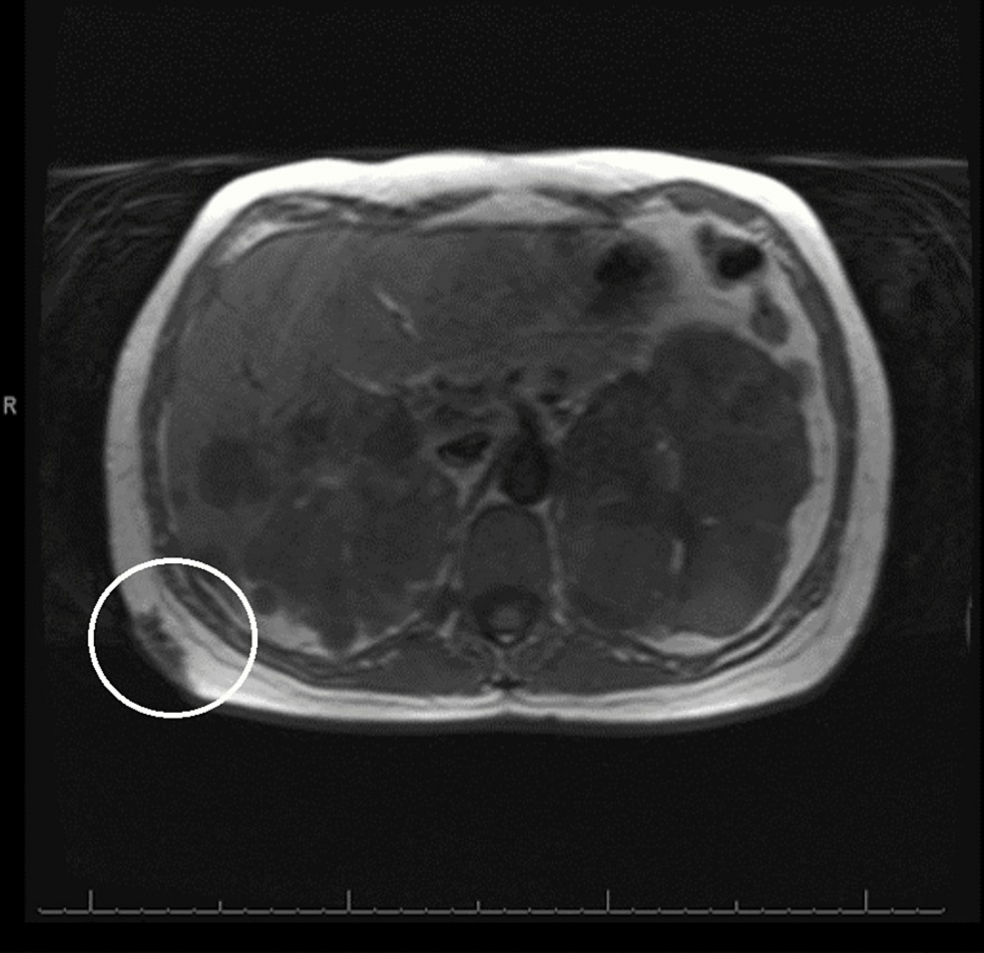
Magnetic resonance imaging of the chest with and without intravenous contrast revealing evidence of polycystic kidney disease affecting the kidneys and liver and a mass in the right-sided posterior chest.
